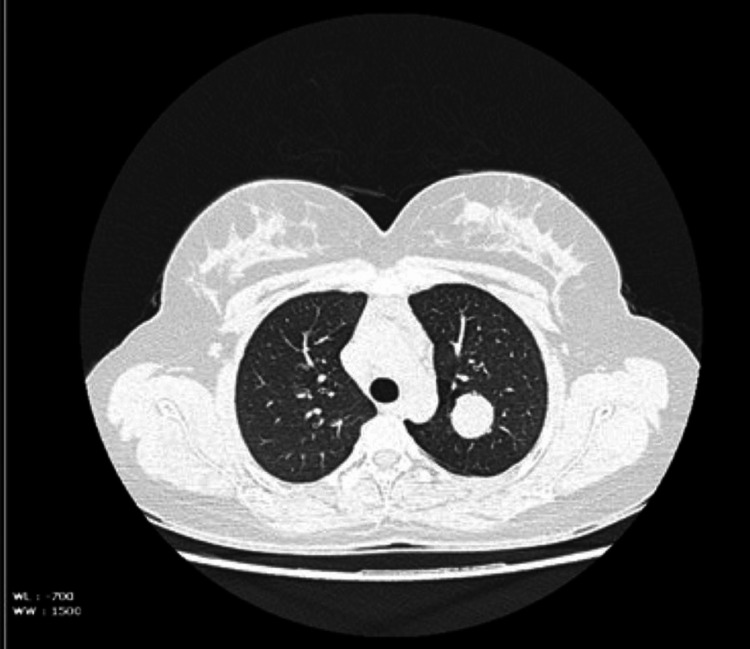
CECT lung shows an intraparenchymal soft tissue density lesion measuring 2.5 x 2.2 x 2.3 cm noted in the apicoposterior segment of the left upper lobe, suggestive of a solitary pulmonary nodule. CECT: contrast enhanced computed tomography
